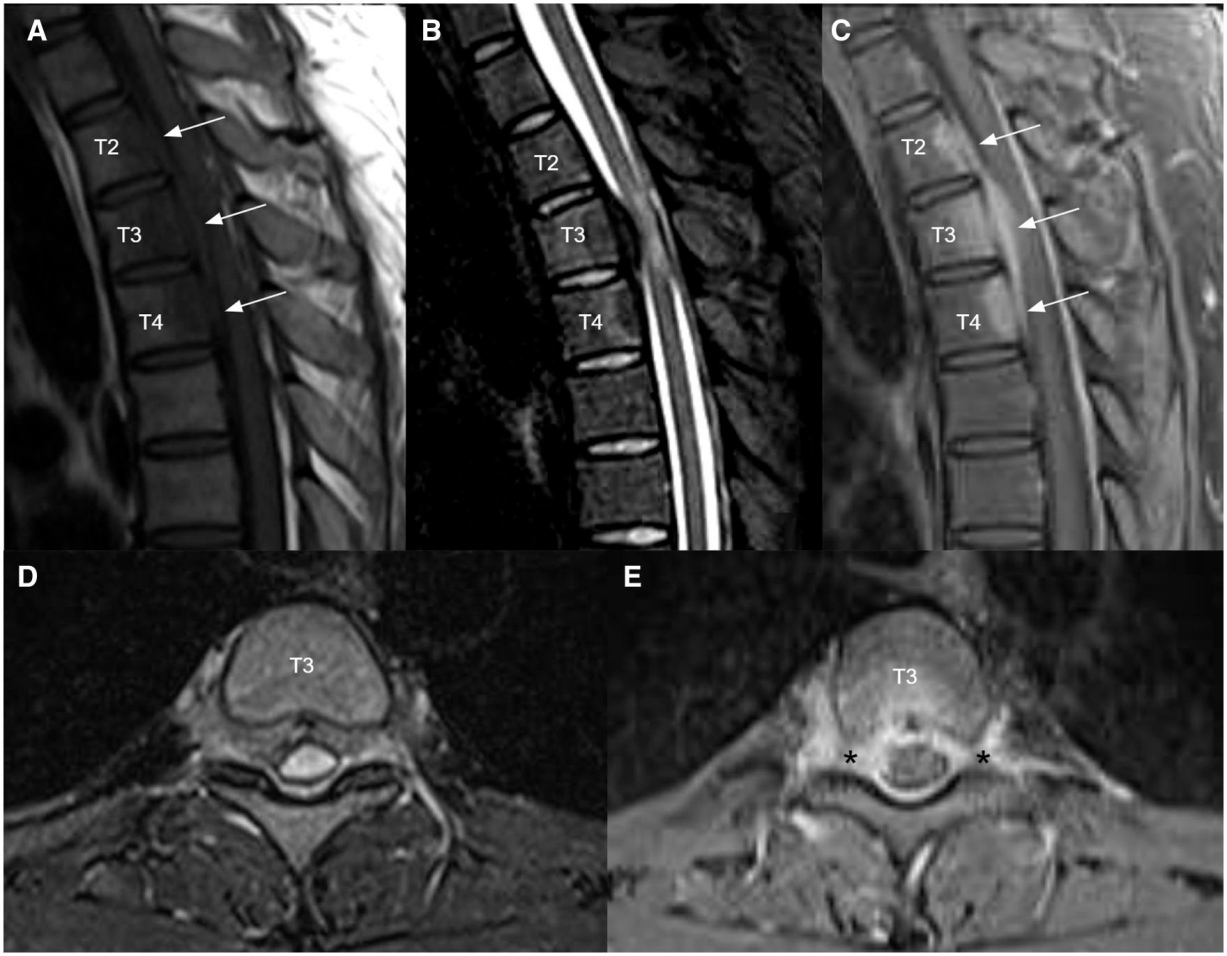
Sagittal T1 weighted (A), T2 STIR (B), and T1 weighted post-contrast (C) MRI images of upper thoracic spine demonstrated an enhancing infiltrative anterior epidural mass at T2 to T4 levels (white arrows) causing mild cord compression mainly at T3 level with cord oedema. Axial T2 STIR (D) and T1 weighted post-contrast (E) at T3 level showed that the epidural mass infiltrates into bilateral T3 lateral recesses and bilateral T3/4 intervertebral foramina (black asterisks). Enhancing lesions were also noted at the posterior aspect of T2 to T4 vertebral bodies (E).
